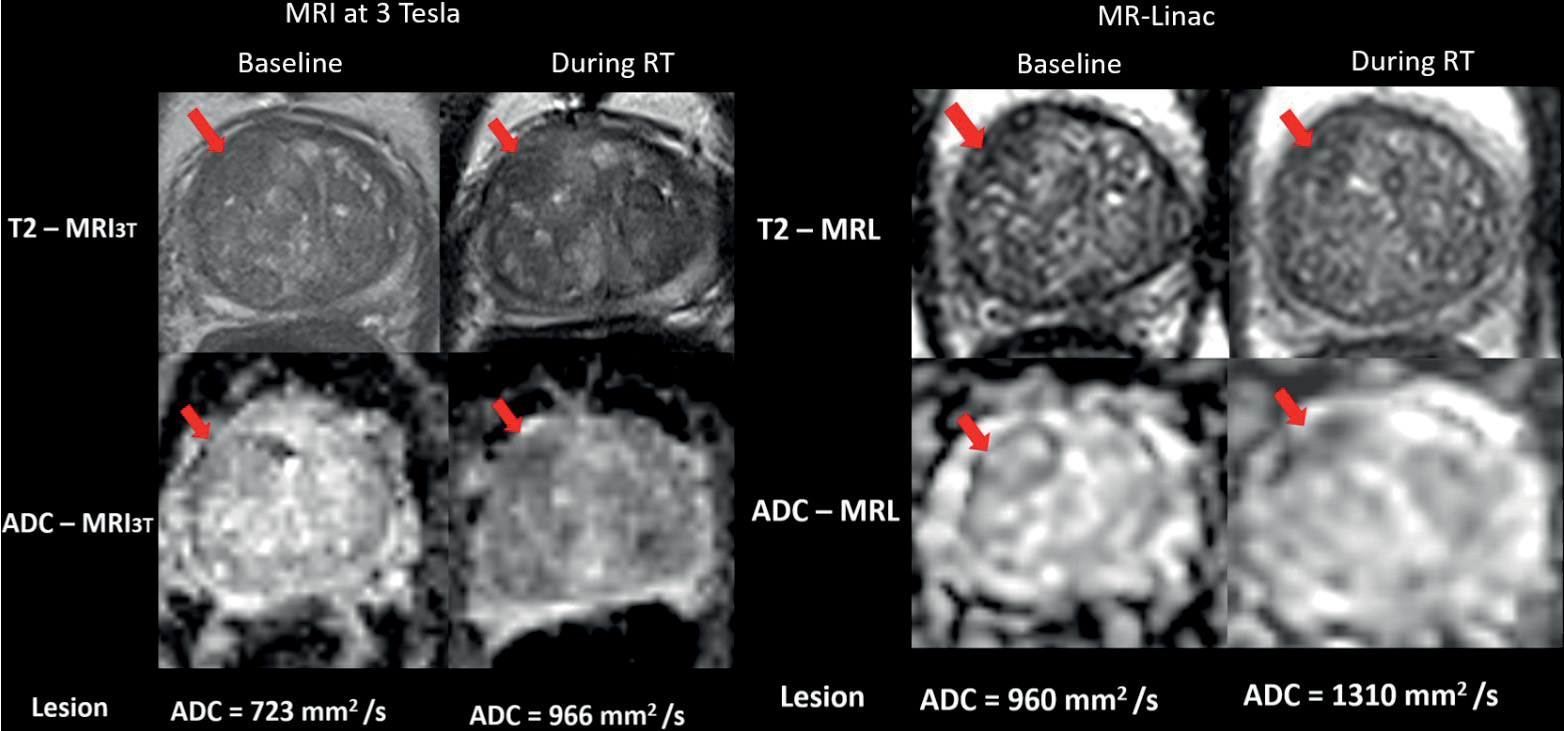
T2-weighted MR images (scan time: 2 minutes) and apparent diffusion coefficient (ADC) maps of a 66-year-old male patient with prostate cancer in the antero-apical region of the transition zone (red arrows) at baseline (left column) and during radiotherapy (right column) as recorded on MR-Linac (MRL) and on standard MRI at 3T (MRI3T). The figure shows similar dynamics of lesion ADC elevation during radiotherapy.
